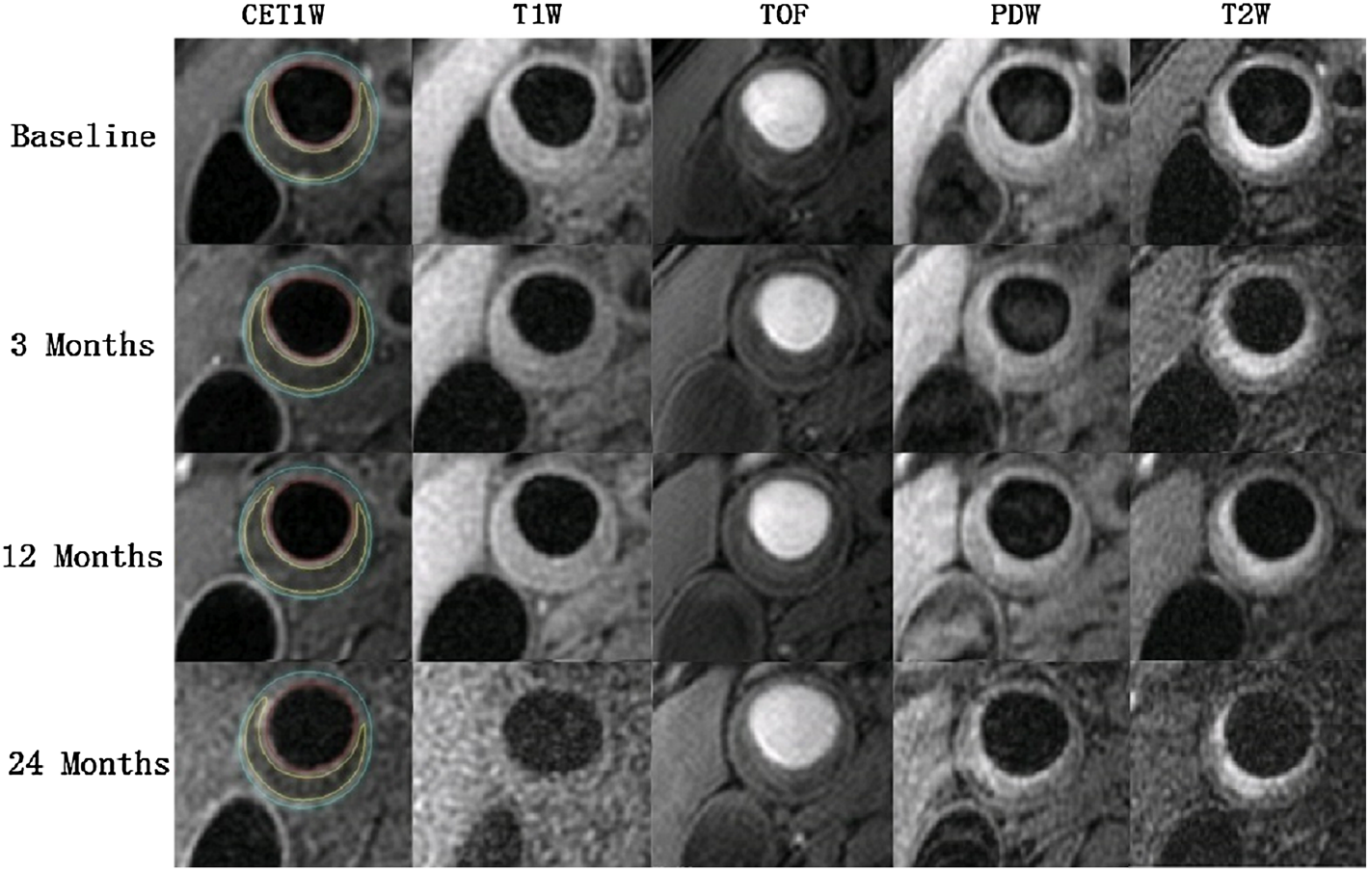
Representative MRIs showing plaque lipid depletion in right common carotid artery over 2 years. The lumen in red, outer wall boundary in blue and lipid content in yellow of the carotid artery were identified and outlined in post-contrast T1W images. LRNC demonstrate isointense on pre-contrast T1W images and TOF, and was detected as non-enhanced areas (relative to surrounding tissues) on CET1W images with the corresponding pre-contrast T1W images used as reference. Regression in LRNC at the same location was found between the baseline, 3 months, 12 months and 24 months MRI scans. MRI = magnetic resonance imaging; LRNC = lipid-rich necrotic core; TOF = time-of-flight; T1W = T1-weighted; T2W = T2-weighted; PDW = proton-density-weighted; CET1W = contrast enhanced T1-weighted.
